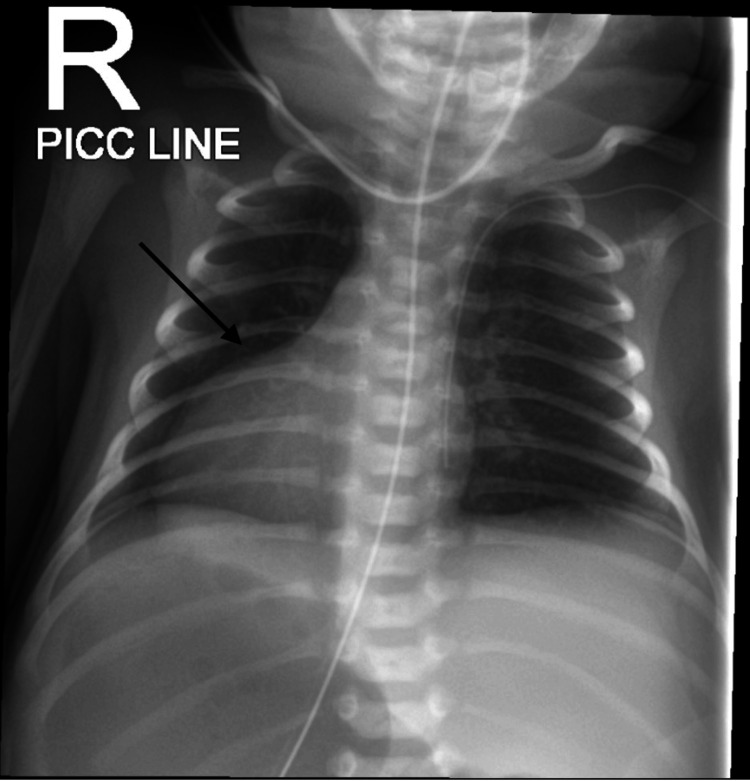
Chest X-ray Frontal Demonstrating dextrocardia.
